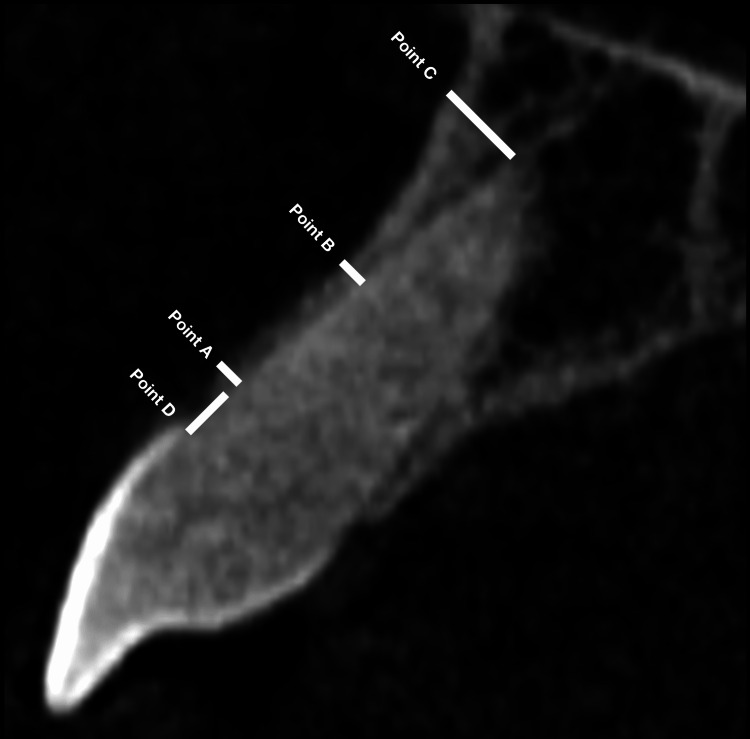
CBCT analysis of the bone thickness of the facial plate and the crestal bone height in a sagittal view from the facial aspect of the tooth root Measurements Point A: from the facial plate at the level of the bone crest to the coronal third of the root; Point B: from the facial plate at the level of the bone crest to the mid-root surface; Point C: from the facial plate at the level of the bone crest to the apical third of the root; Point D: from the cementoenamel junction (CEJ) to the alveolar crest CBCT: cone beam computed tomography
